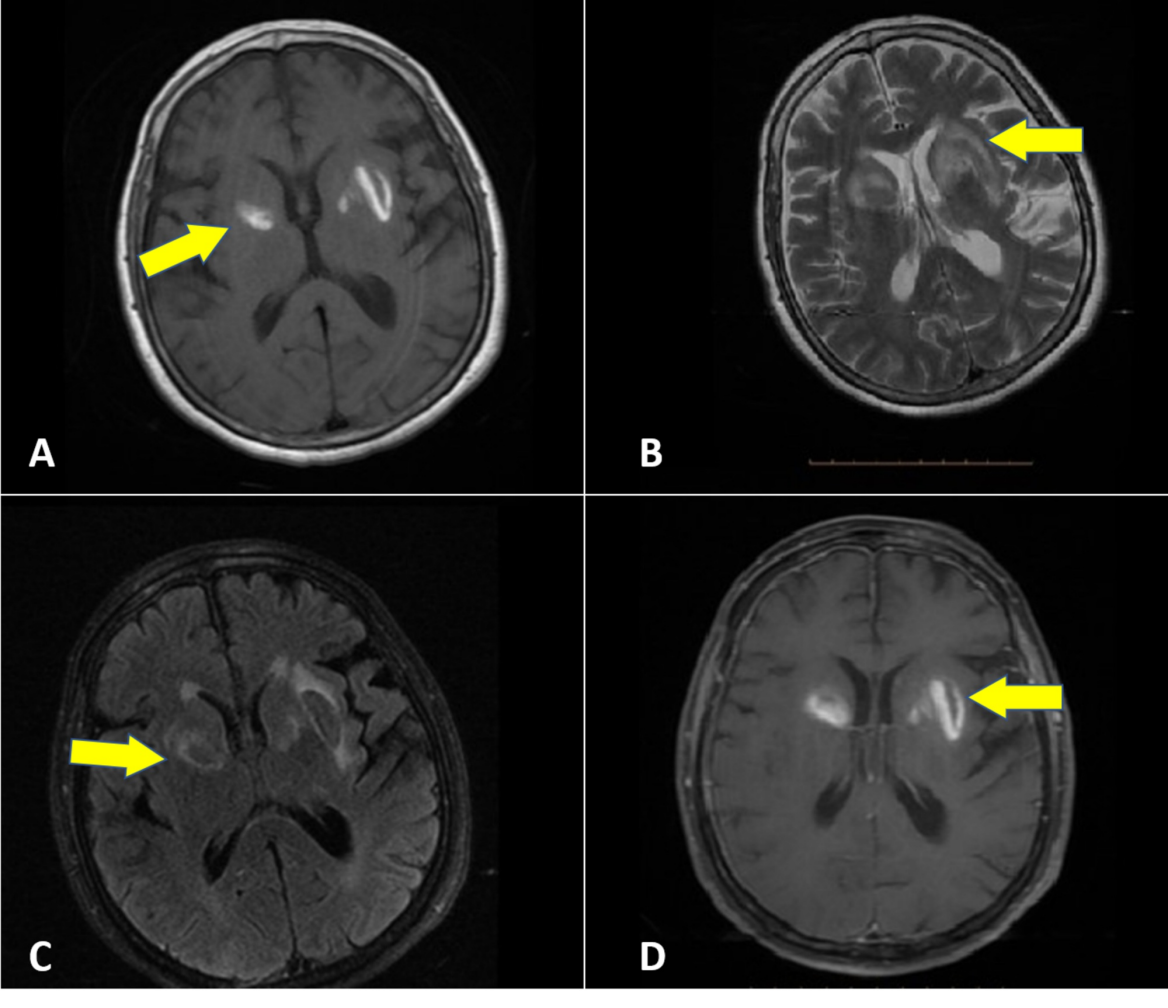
Magnetic resonance imaging T1 (A), T2 (B), fluid-attenuated inversion recovery (C), and gadolinium+ (D) showing the signal change in bilateral basal ganglia matching a bleeding event
